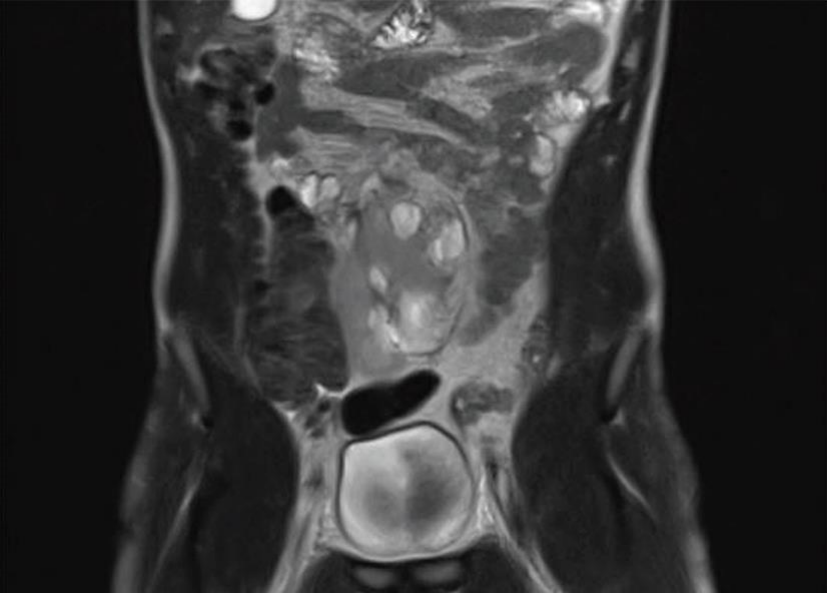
Magnetic resonance imaging-ectopic pelvic right kidney.
